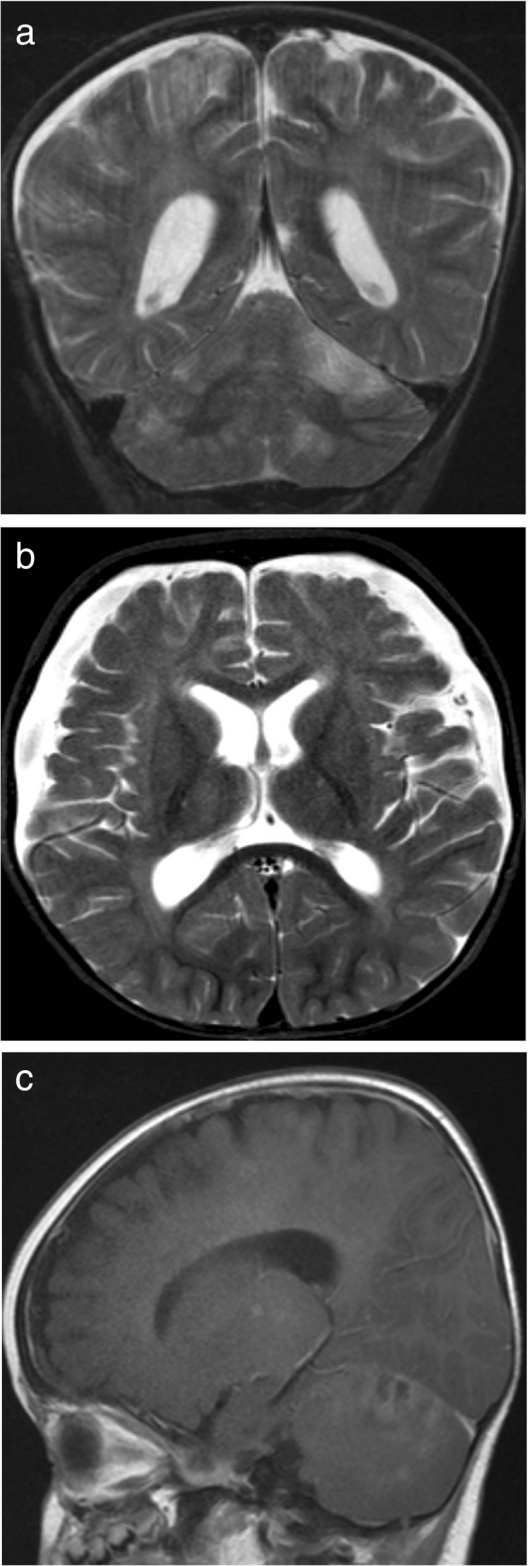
Axial (a) and Coronal (b) T2-weighted images and Sagittal (c) Post-contrast T1 weighted images of the brain MRI in P2, showing multiple focal lesions in the cerebral hemispheres and cerebellum involving both grey and white matter with contrast enhancement. Bilateral subdural collections were also present
